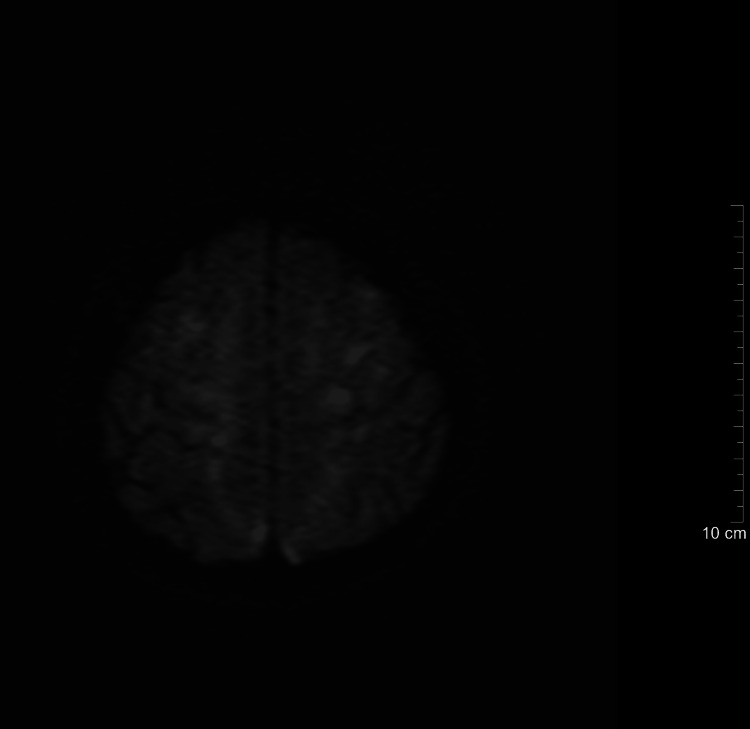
Post-treatment brain MRI (diffusion-weighted imaging, Day 7). This diffusion-weighted MRI obtained seven days after initiation of intravenous antimicrobial therapy shows marked reduction in restricted-diffusion lesions compared to baseline.
